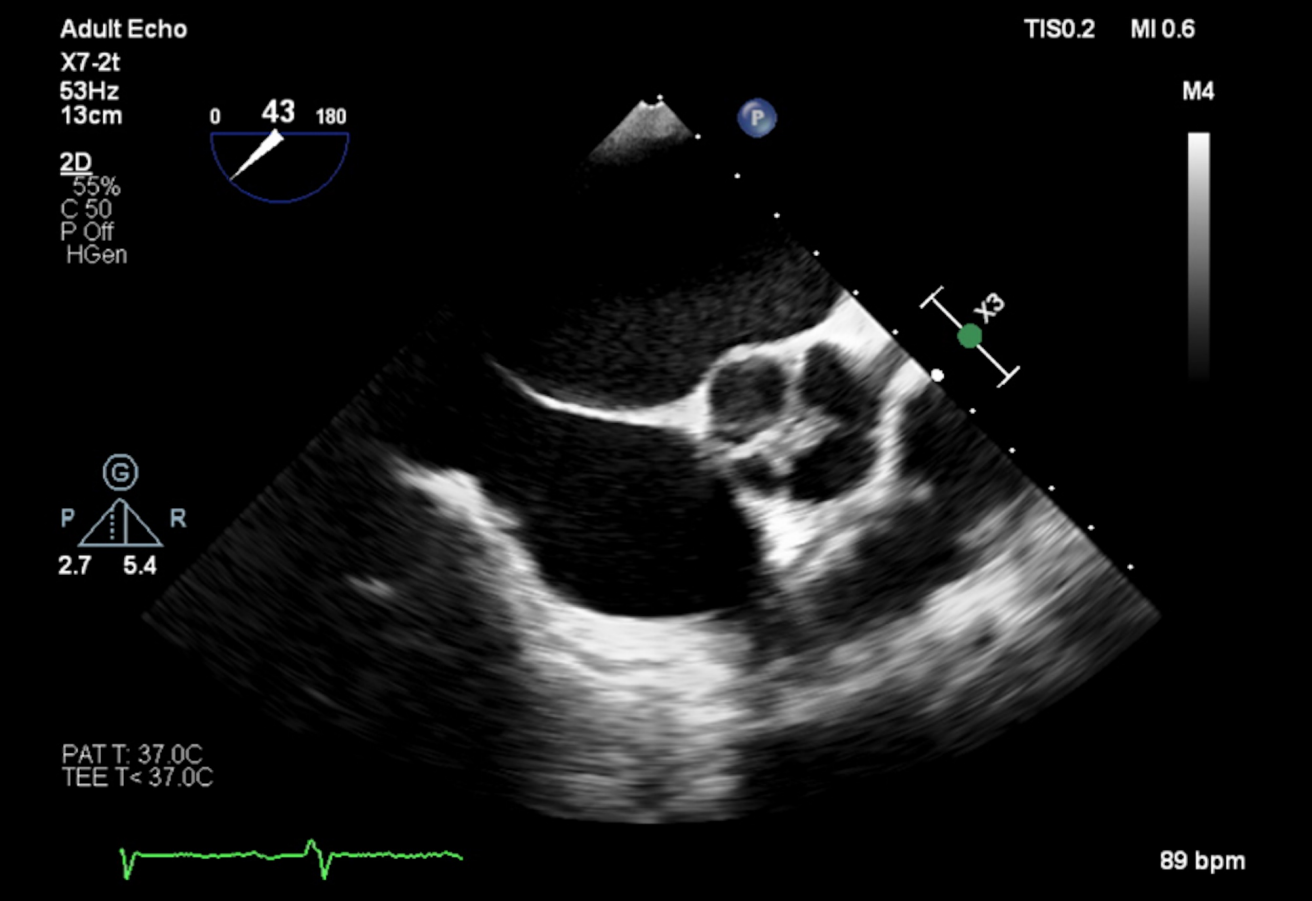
Transesophageal echocardiogram The TEE showing an aortic valve with four cusps. This QAV is classified as “Hurwitz and Roberts” type B, with three cusps almost identical in size and one smaller cusp. QAV: Quadricuspid aortic valve; TEE: Transesophageal echocardiography.
